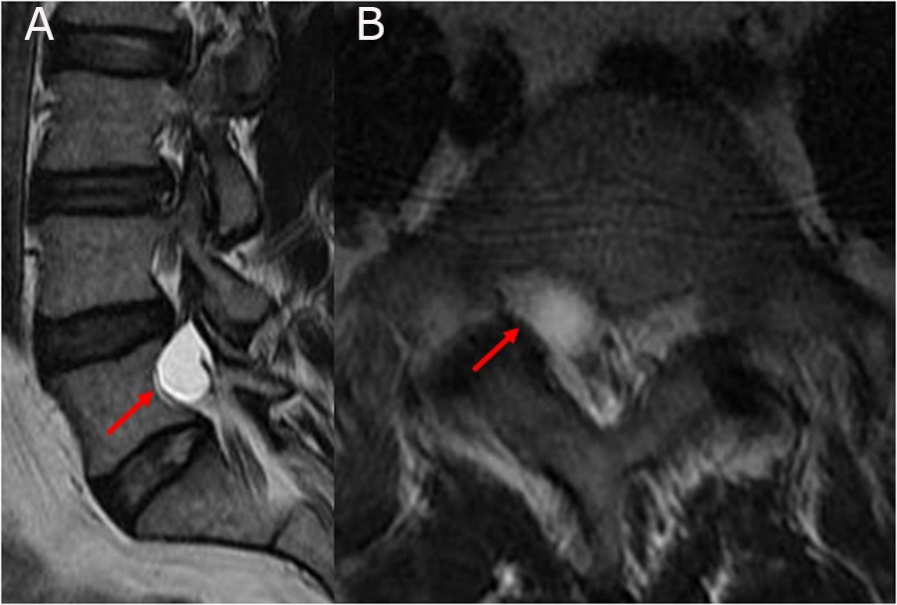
A) The right parasagittal T2W image showing fluid-filled cyst involving the fifth lumbar (L5) nerve root (red arrow). B) The axial T2W image showing the foraminal location of the cyst (red arrow). Note: The image quality is poor because the patient was unable to lie still during the magnetic resonance imaging (MRI).
